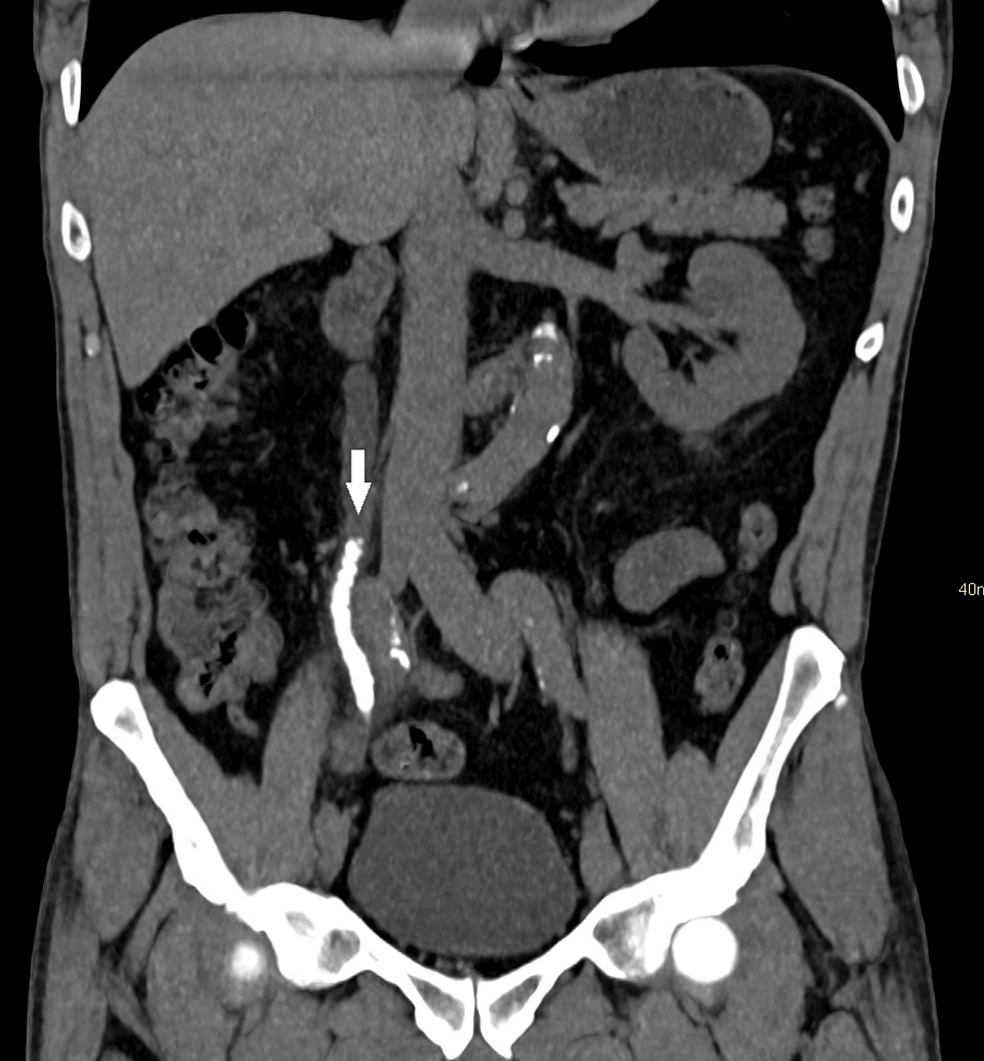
Computed tomography (coronal axis) showing right-side steinstrasse and ipsilateral ureteral dilation (arrow).
